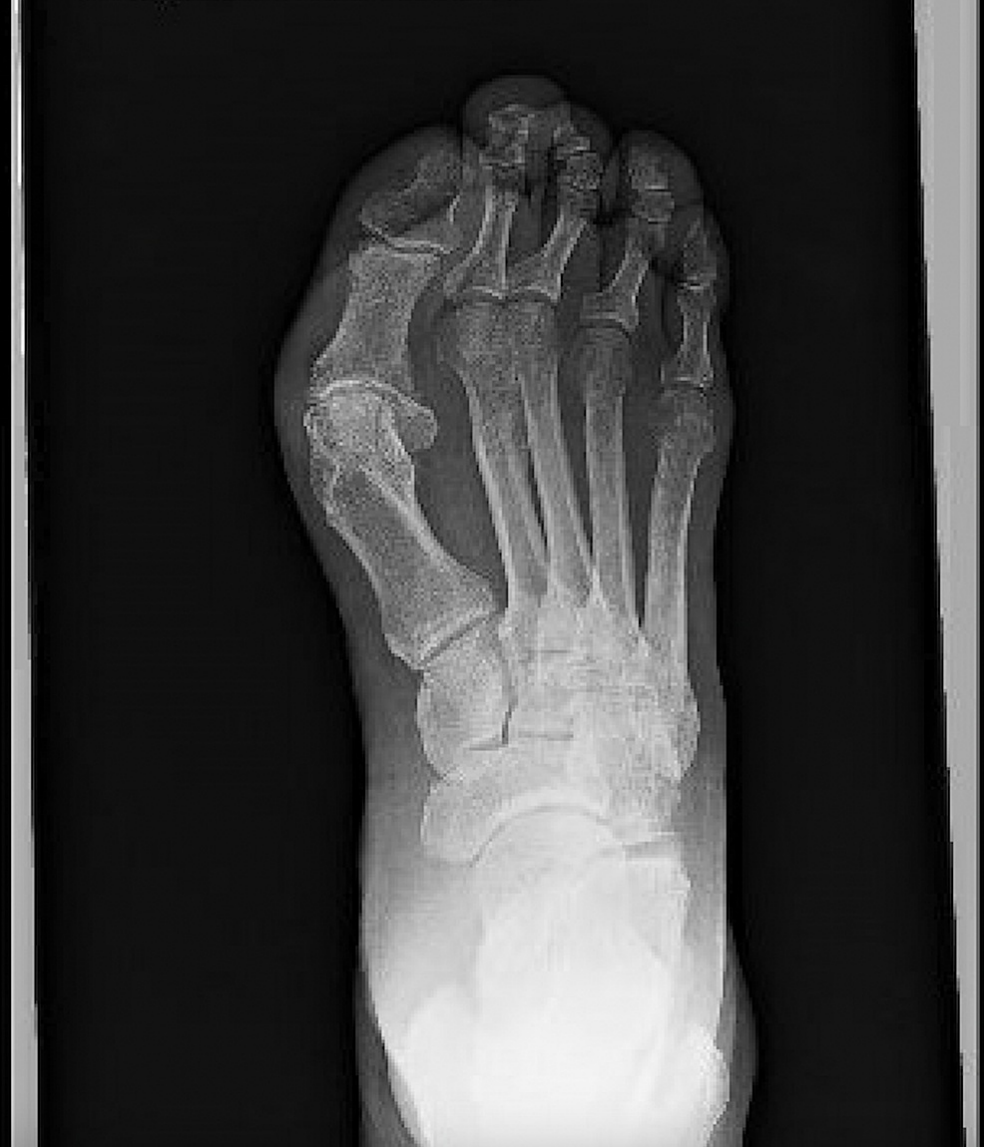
X-ray of the right foot which demonstrated severe degenerative changes of the first metatarsal phalangeal joint without evidence of acute osteomyelitis.
